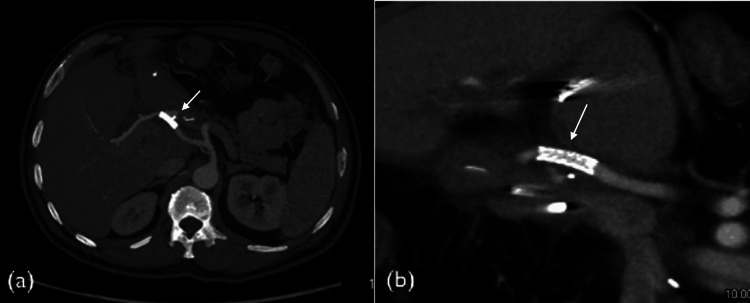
Early follow-up CT scan in a patient with a common hepatic artery stent graft. Maximum intensity projection images in the axial (a) and sagittal (b) planes obtained two months after implantation of a 5 mm × 22 mm BeGraft stent (white arrows) demonstrate patency of the stent.
